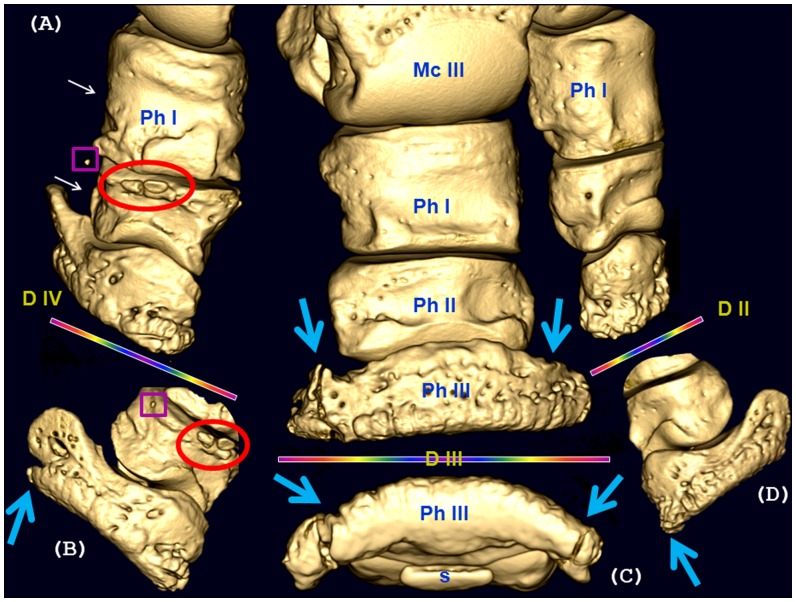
Bone pathology of the distal right front limb in rhinoceros 3. Dorsal aspect of the distal autopodium (A) and close-up computed tomographic images of distal phalanges (Ph) from lateral (D IV) digit (B), middle (D III) digit (C) and medial (D II) digit (D) showed multiple osteopathologies. Distal phalanges (Ph III) of each digit present fractures (large arrows) characterized as follows: medial digit (D II) – complete, with dorso-distal triangular fractured fragment of bone; central digit (D III) - severe, comminuted fracture of the lateral palmar process, with two main fragments (20×30×10 mm and 10×20×10 mm) accompanied by callus formation and also severe fracture of the medial palmar process with one fragment; lateral digit (D IV) - chip fracture of the distal part of lateral palmar process. Other pathologic changes observed on D IV include: small mineralized body (2 mm diameter, A, B-rectangle) between the first phalanx (Ph I) and the second phalanx (Ph II); two medium sized osseous bodies (10 mm length, A, B-circle) situated dorso-proximally to Ph II; dorso-medial amorphous periosteal reaction on the proximal half of Ph II; moderate bone remodelling of Ph I and Ph II (small arrows). The small sesamoid (s) of D III is intact.
